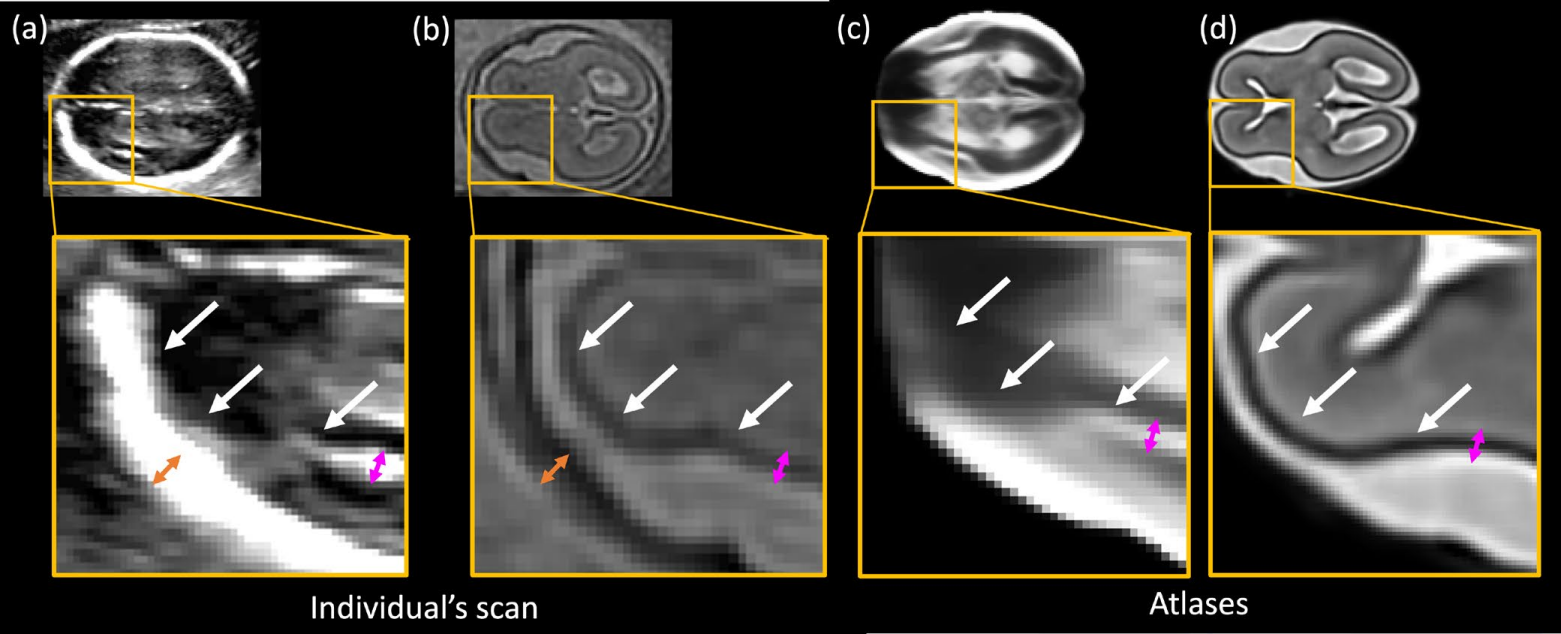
Same‐day US (a) and MRI volume (b) at 21 GW and the atlas volumes from US (c) and MRI (d) at the same age (Namburete et al. 2023; Uus, Kyriakopoulou, et al. 2023). The same size arrows in the same position have been added to each. The white arrows show the cortical plate boundary, the orange indicates the skull thickness based on the MRI, showing the US skull appears thicker and the pink arrows show the CoP ribbon, modelled on the US volume. In the US volume, regions of the pericerebral space, that are visible in the MRI, are missing. This study investigates the agreement of image‐derived phenotypes (IDPs) from eight fetal brain structures derived from same‐day MRI and 3D US volumes. Strong agreement was observed for the CSP, Th, CB, WMDGM, whereas systematic biases were revealed for ICV and CoP.
